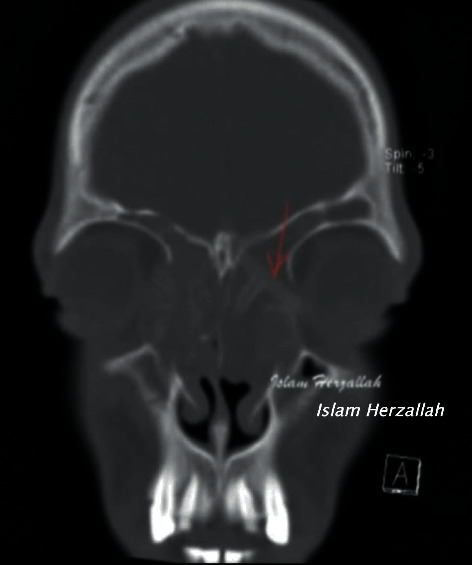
Preoperative CT scan showing migrating frontal sinus stent.
